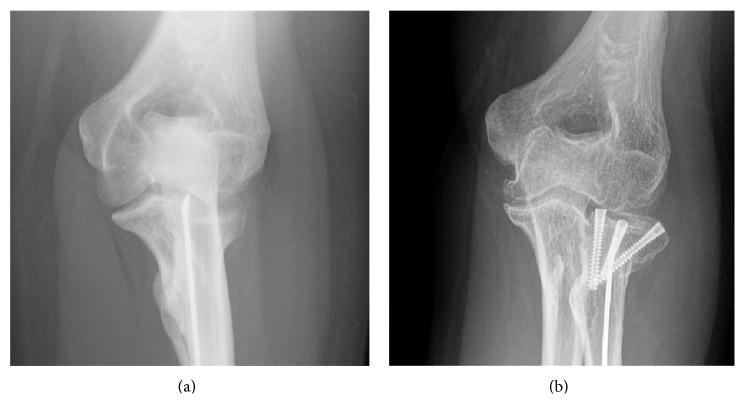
(a) Preoperative anteroposterior X-ray showing the radial neck malunion. (b) Postoperative anteroposterior X-ray showing the radial head fixation.
